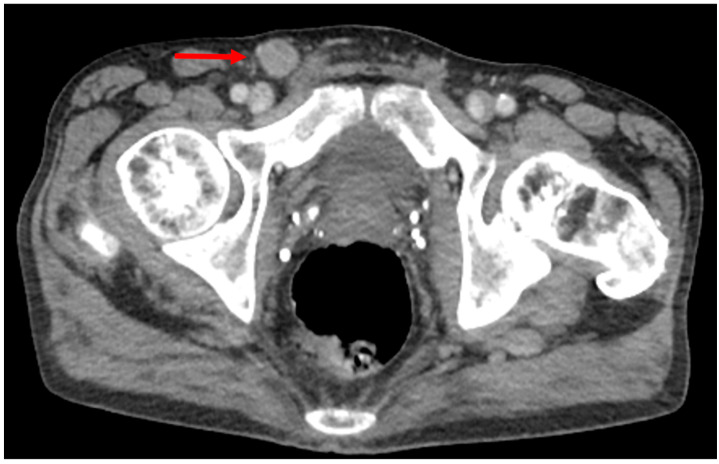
55-year-old male with newly diagnosed penile cancer presents for initial staging CT of the abdomen and pelvis. Axial contrast enhanced CT through the level of the pelvis demonstrates an abnormally rounded, enlarged right inguinal lymph node (arrow) that was confirmed to be metastatic at time of surgery.
